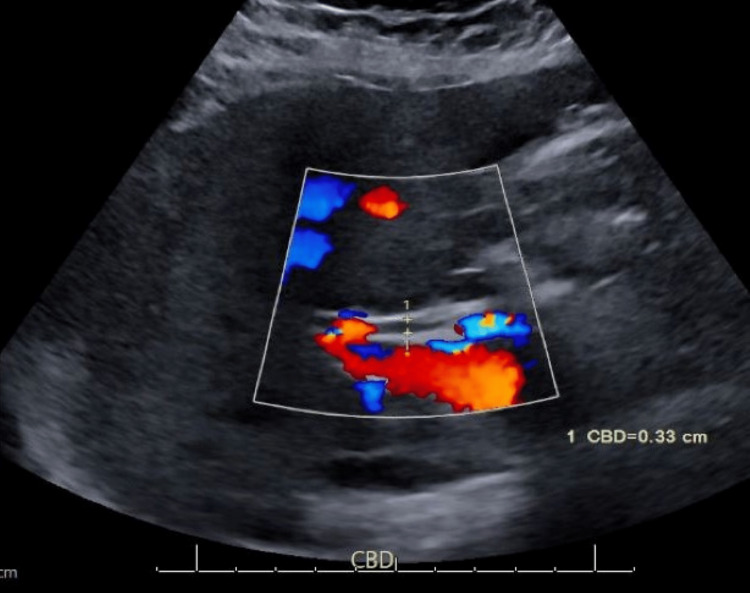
Ultrasound of the abdomen showing normal common bile duct diameter (0.33 cm)
